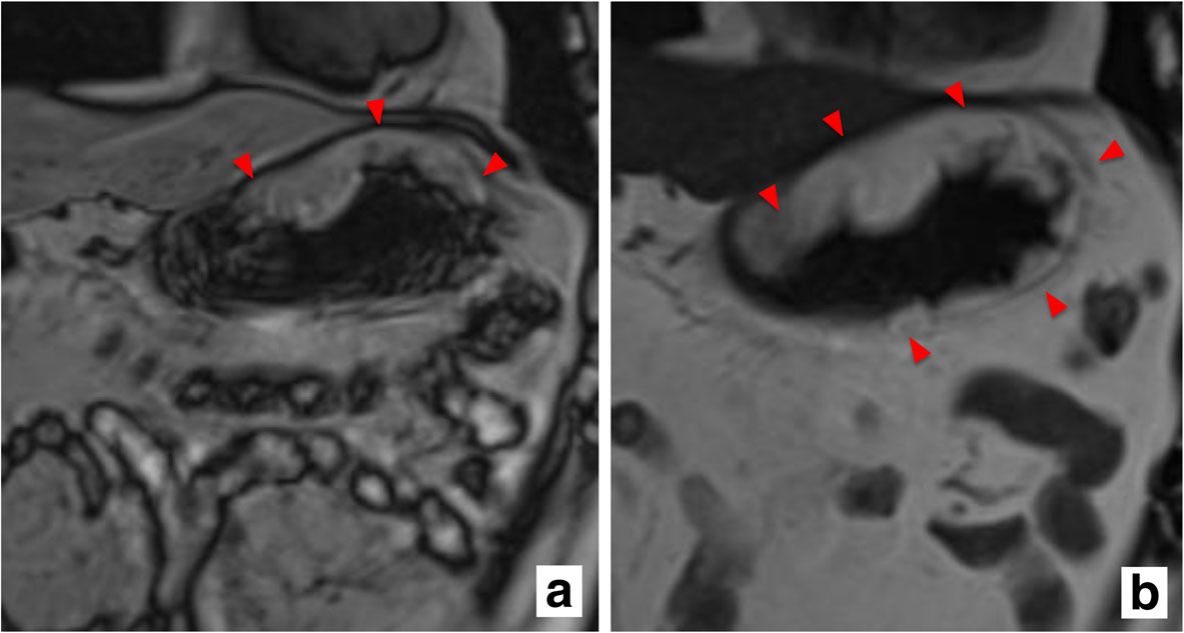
Findings on T1- and T2-weighted MRI. T1- (a) and T2-weighted (b) MRI findings are shown. There is a high-intensity lesion in the submucosa with a fat-containing mass on the entire wall of the gastric antrum and body, but excluding the lesser curvature (red triangle). Abbreviations: MRI magnetic resonance imaging
